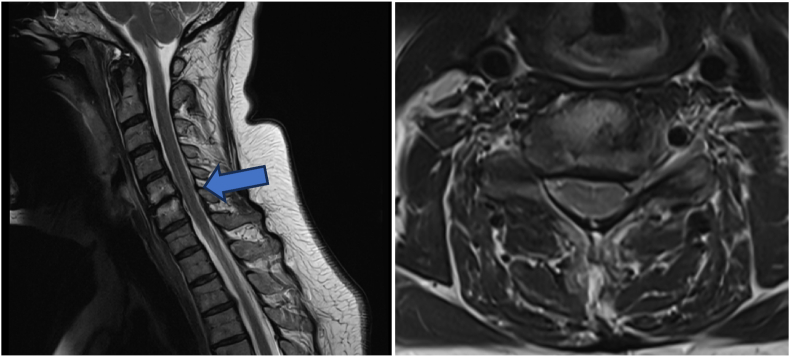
MRI at one-year post-op revealed a very subtle cord signal change (arrowhead). A T2W axial cut at the level of C5-6 shown on the right.
